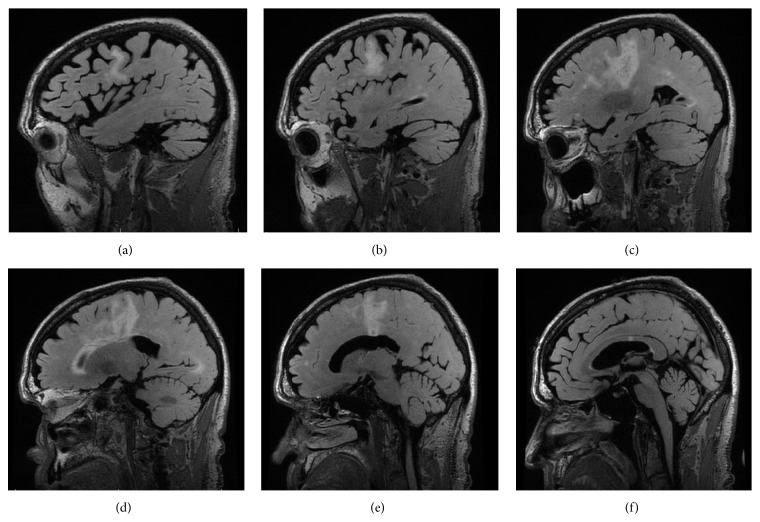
Sagittal images of MRI of the brain with T2-weighted FLAIR hyperintensities. Images progress from left to right from (a) through (f). Areas of demyelination correspond anatomically with axial images from Figure 1.
